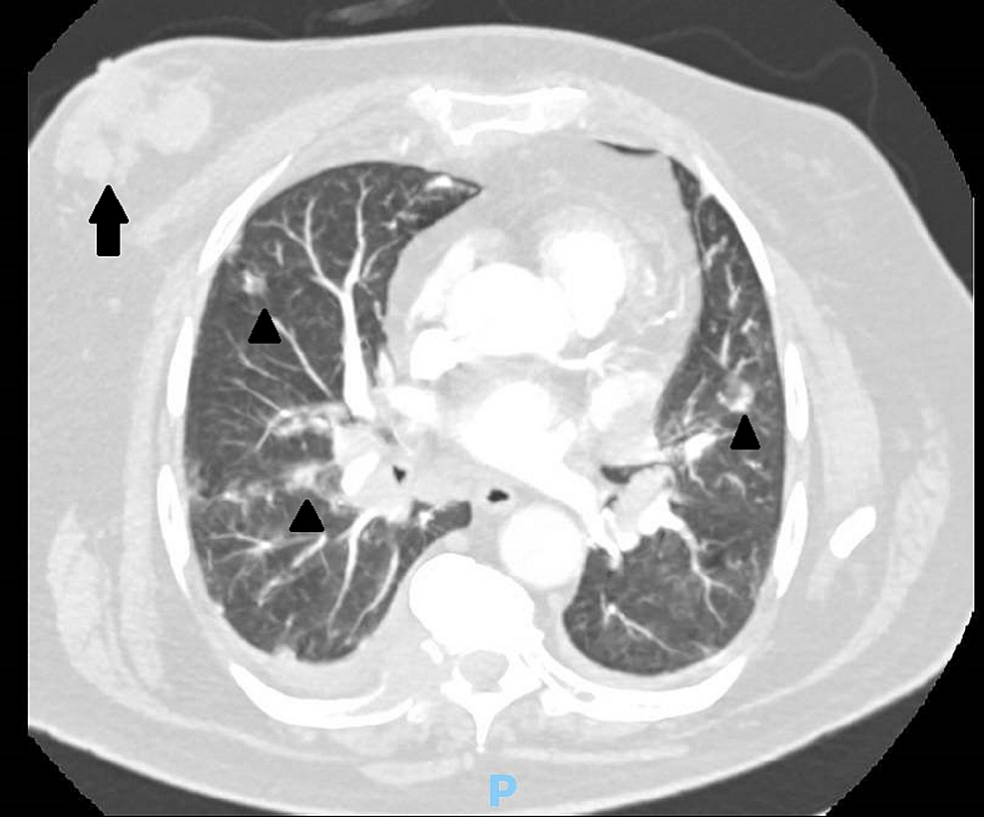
Chest CT angiography showing right retroareolar glandular tissue enlargement (arrow) and bilateral pulmonary nodules (arrowheads)
